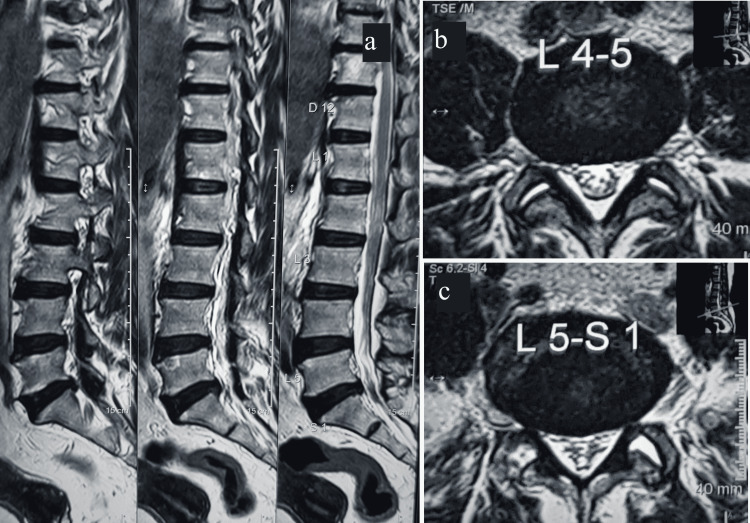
Magnetic resonance imaging of the lumbar spine showing discoid degenerative changes in sagittal section (a), nerve root compression at L4-L5 level (b), and nerve root compression at L5-S1 disc level (c).
